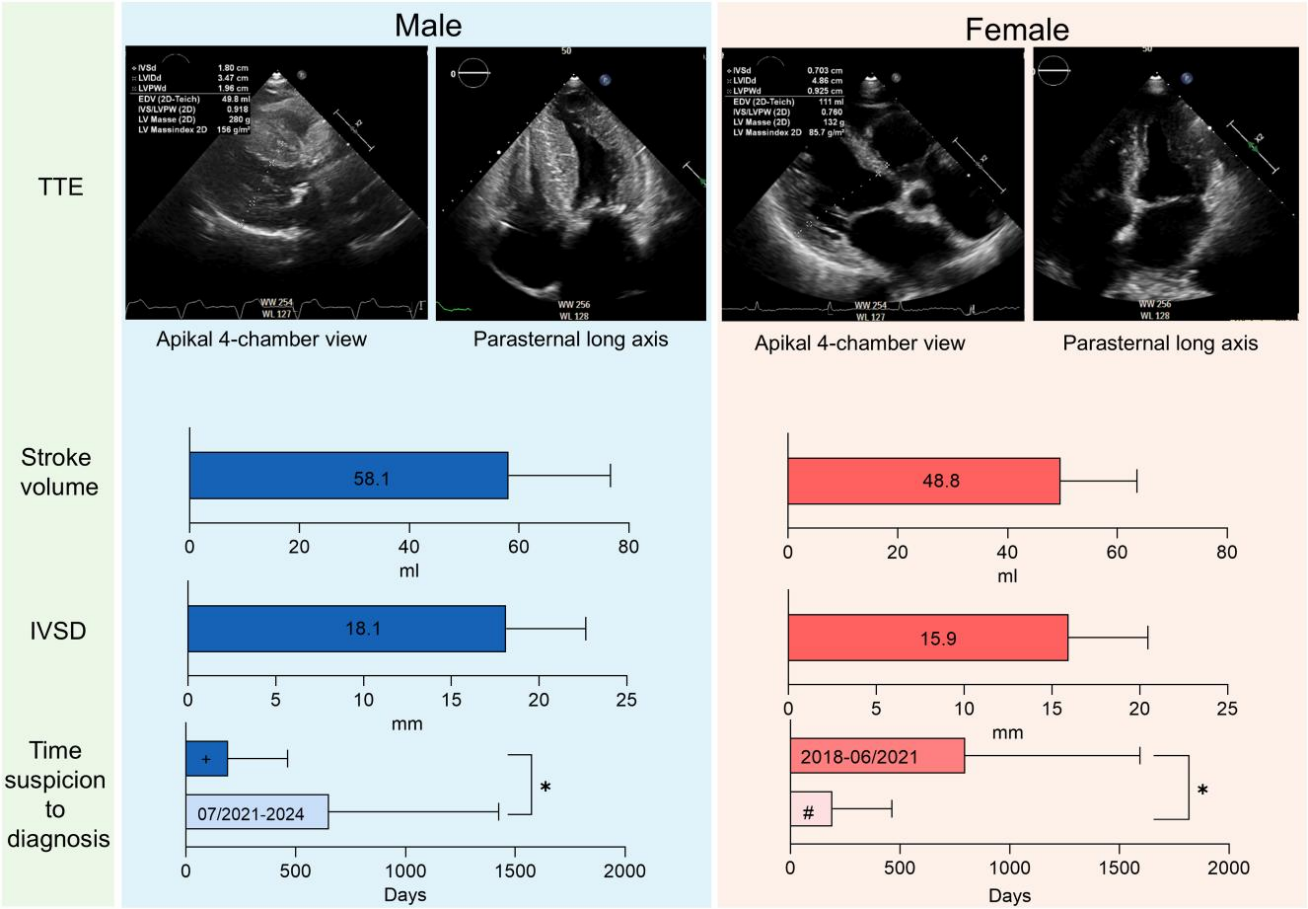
Echocardiographic and time from suspicion to diagnosis differences between women and men. IVSD, interventricular septum diameter; TTE, transthoracic echocardiography; P* < 0.05; ^+^2018–06/2021; ^#^07/2021–2024. Mean ± SD.
